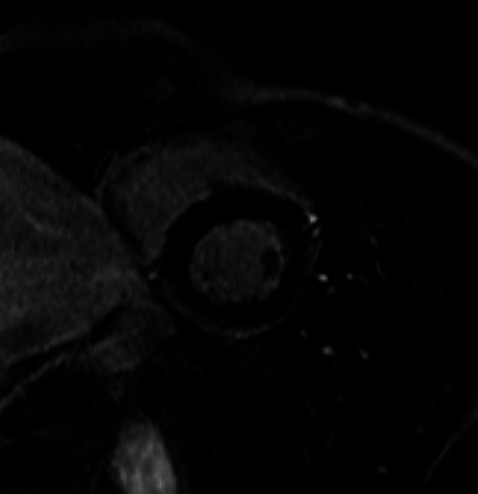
MRI analysis. Precontrast images: on stir T2w images, absence of myocardial areas with signal hyperintensity compatible with oedema.
